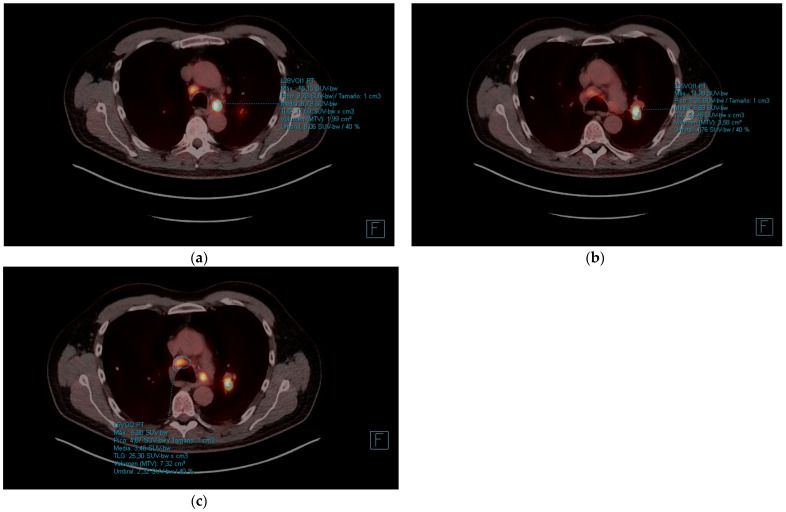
Standard PET-CT scan. Multiple hypermetabolic adenopathies, the most representative being left paratracheal (a) (SUVmax 15), left hilar (b) (SUVmax 11.9), and subcarinal (c) (SUVmax 5.8). Biopsy showed a non-necrotizing lymphogranulomatous reaction.
